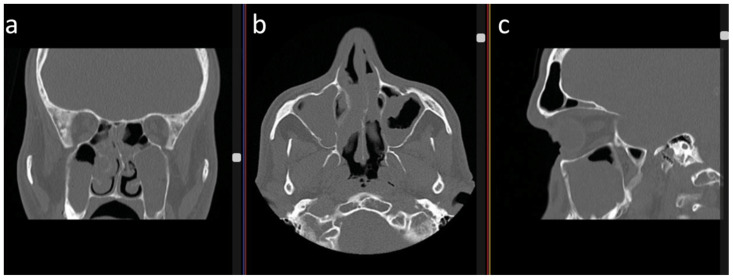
CT scan in three spatial planes in a patient suffering from CRS. (a) Coronal reconstruction with opacification of the maxillary sinus and the ethmoid sinus bilaterally on both sides. (b) Axial reconstruction; when viewed together with A, it can be observed that there is a subtotal obstruction in both maxillary sinuses due to secretion and mucosal swelling. (c) Sagittal reconstruction; the frontal sinus is largely unaffected. There is discrete residual ventilation in the cranial part of the maxillary sinus.
